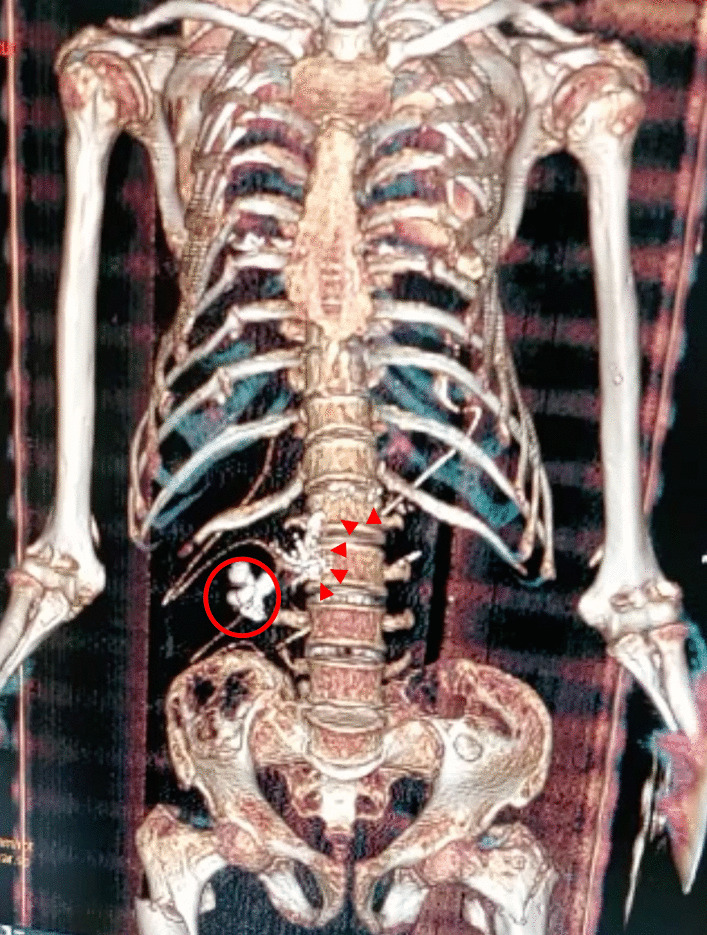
CT 3D bone reconstruction. Staghorn calculus (red circle) and pancreatic calcifications (arrowheads)
